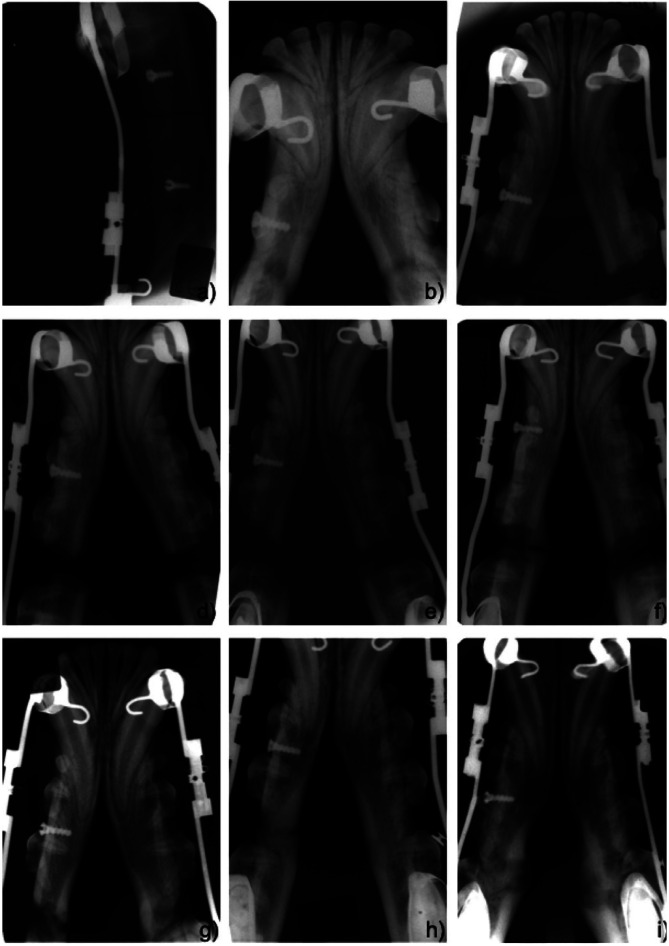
Radiographic healing patterns: immediate postoperative radiograph (a), one week after osteotomy (b); ten days after distraction (c); with two weeks of the consolidation period (d); with four weeks of the consolidation period (e); with six weeks of the consolidation period (f); with eight weeks of the consolidation period (g); with ten weeks of the consolidation period (h); and, with twelve weeks of the consolidation period (i).
